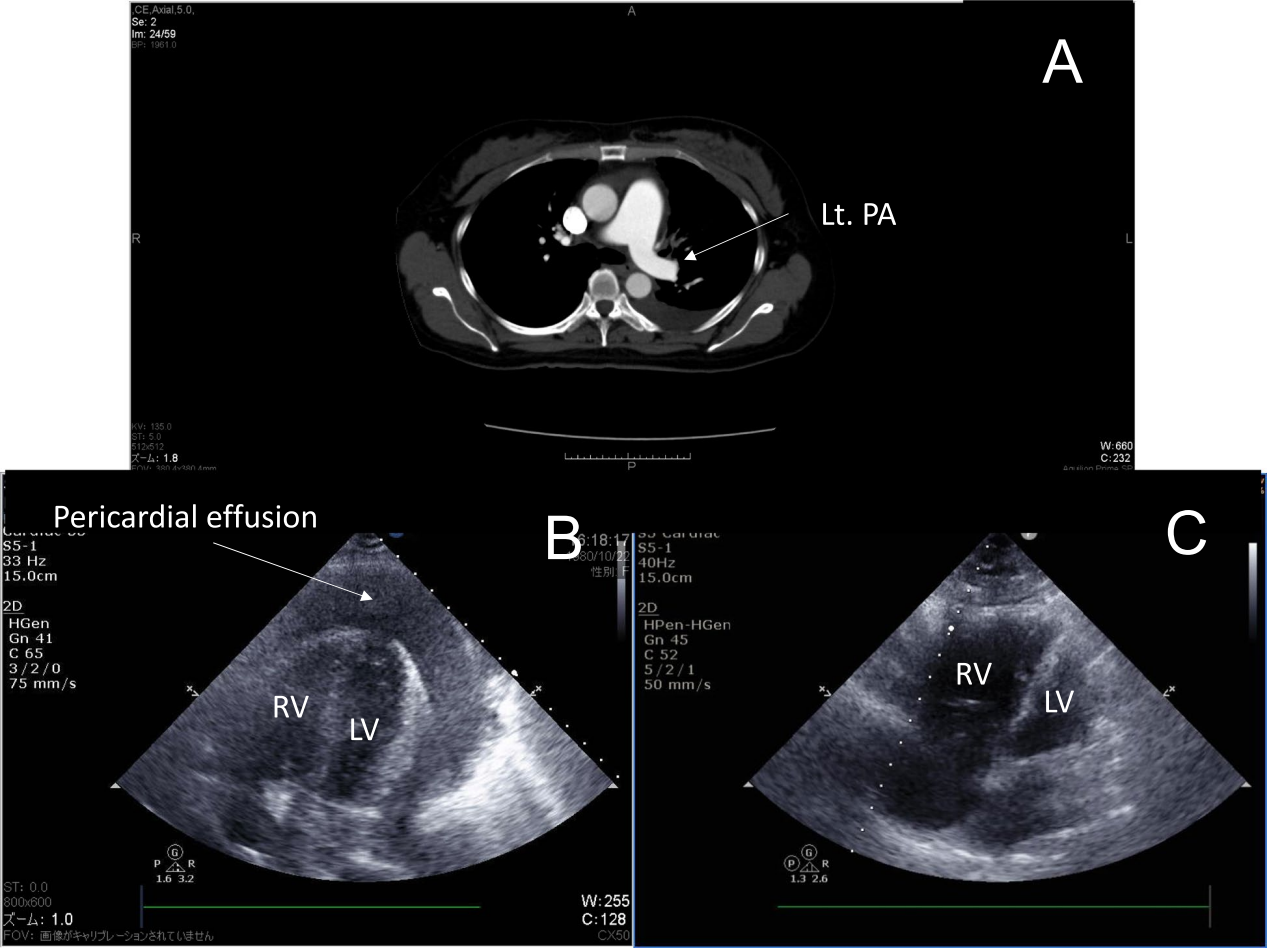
Images of CT pulmonary angiogram and transthoracic echocardiography. A CT pulmonary angiography demonstrates pulmonary embolism in the left main pulmonary artery (arrow head) a day before pericardiocentesis. B and C Apical four chamber view on transthoracic echocardiography. Massive pericardial effusion before pericardiocentesis (B); remarkably dilated right ventricle and decompressed left ventricle after removal of pericardial effusion (C). Lt.PA; left main pulmonary artery, RV; right ventricle, LV; left ventricle
